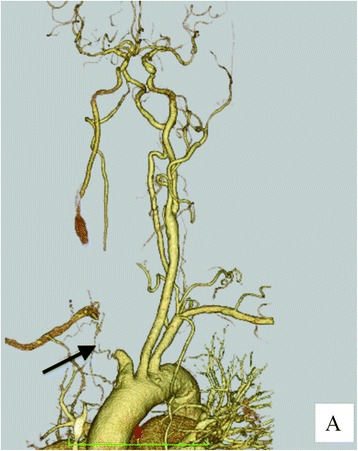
CT angiography of neck vessels. (*Arrow*) Ligation of innominate artery. The distal right common carotid and right subclavian arteries were enhanced by collateral flow through the left common carotid artery and the circle of Willis
